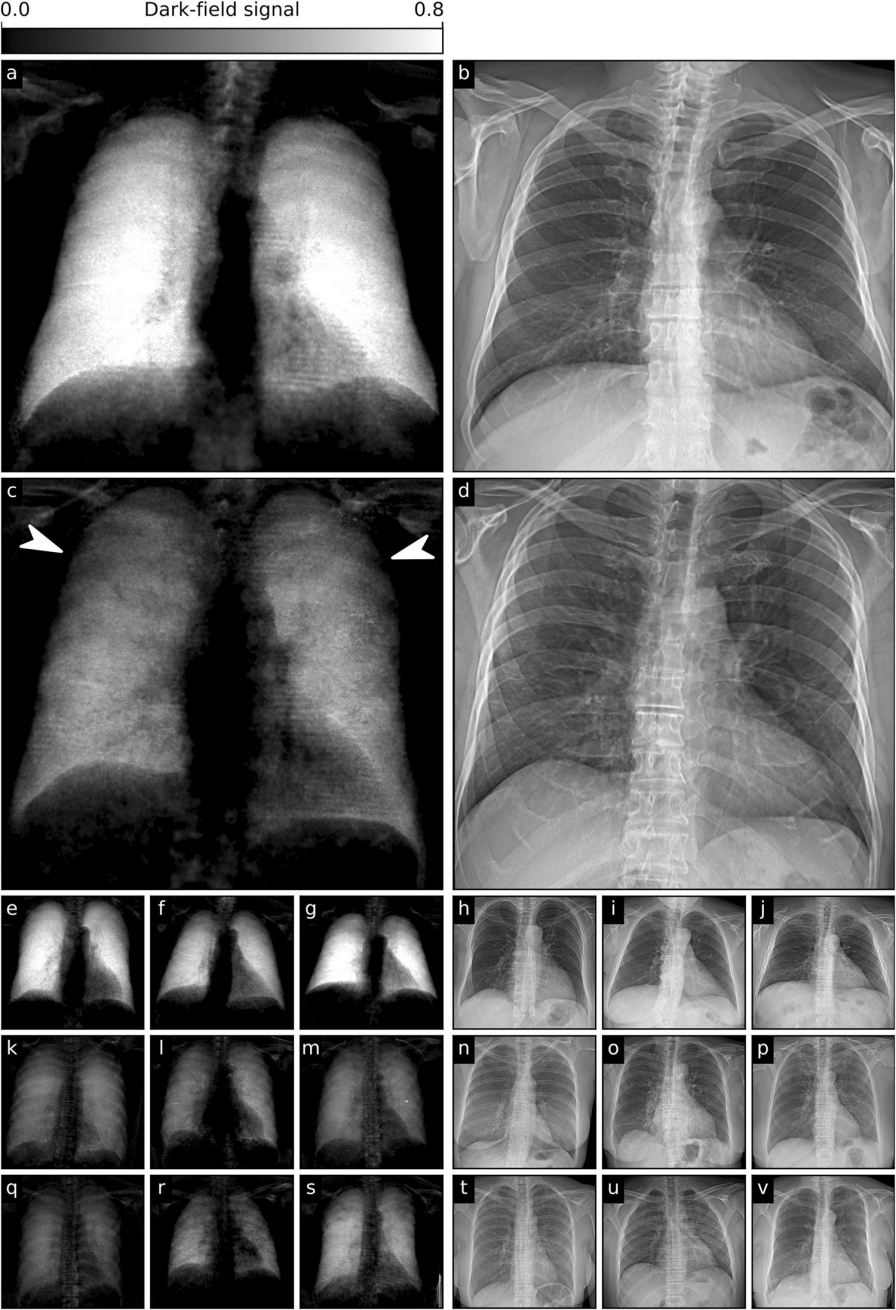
Dark-field and conventional chest X-rays of healthy and COVID-19-infected subjects. a Dark-field and b conventional (attenuation-based) chest radiographs of a healthy subject. The dark-field radiograph exhibits a strong, homogeneous dark-field signal. The respective attenuation-based image shows no apparent pathology. c Dark-field and d attenuation-based chest radiographs of a patient infected with COVID-19. Compared to the healthy subject, the infected patient shows an overall decrease of signal intensity. While the signal of the healthy subject is homogeneous, the dark-field signal of the infected patient appears inhomogeneous and patchy, especially in the periphery of the lung (arrowheads). e–g Additional exemplary dark-field radiographs of healthy subjects, h–j, respective attenuation-based radiographs. k–v More exemplary dark-field and respective attenuation-based images of patients infected with COVID-19. k–m, Dark-field images with a generally reduced signal intensity. q-s, Dark-field images with a rather inhomogeneous, patchy texture, predominantly in the periphery. n–p and t–v respective attenuation-based images in comparison, in which it is rather difficult to impossible to detect COVID-19-pneumonia.
